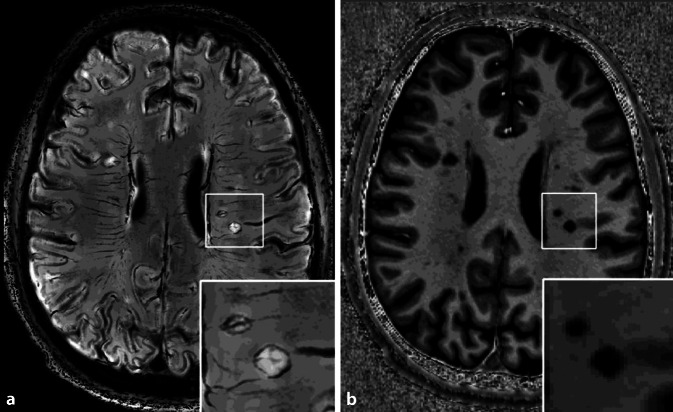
7‑T FLAIR-SWI (a) and MP2RAGE (b) images show cerebral white matter lesions in a 38-year-old male patient with relapsing-remitting multiple sclerosis with a disease duration of 3 years, an Expanded Disability Status Scale of 2.5, and a Multiple Sclerosis Severity Score of 6. Image a shows FLAIR-hyperintense lesions with (paramagnetic rim lesions [PRLs]s) and without (non-PRLs) SWI-hypointense rims. Corresponding MP2RAGE lesions (b) show increased T1-hypointensity in PRLs compared to non-PRLs, indicating the prominent tissue destruction of PRLs. Two PRLs with a central vein sign are magnified in the white rectangle in (a) and (b)
